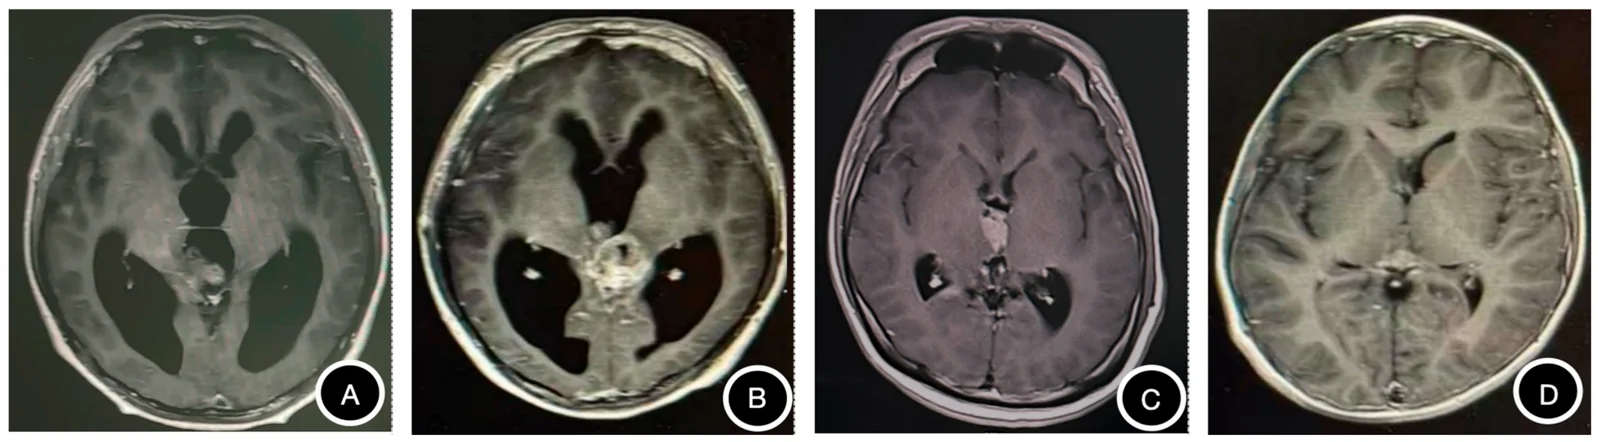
Contrast-enhanced axial T1-weighted magnetic resonance images. (A) Case 1: irregular cystic–solid mass in the posterior third ventricle/pineal region, with strong enhancement of the solid component and cyst wall. (B) Case 2: mixed-signal posterior third ventricular mass with heterogeneous nodular and patchy enhancement. (C) Case 3: cystic–solid third ventricular mass with marked heterogeneous enhancement. (D) Case 4: pineal region and third ventricular mass with mild-to-moderate heterogeneous enhancement.
